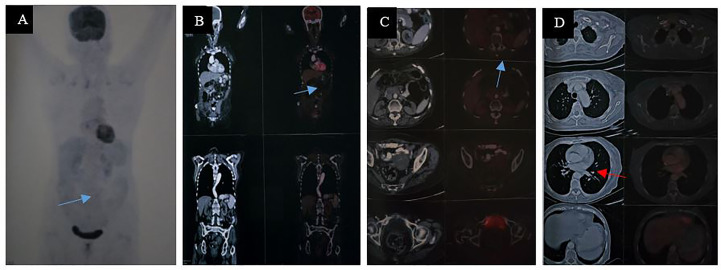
Repeat PET/CT, maximum intensity projection (MIP) on 16 January 2025 was in favor of the resolution of a previous FDG-avid abdominal wall focus [(A–C) the site was marked by a blue arrow] and the lung nodules [(D) marked by a red arrow].
